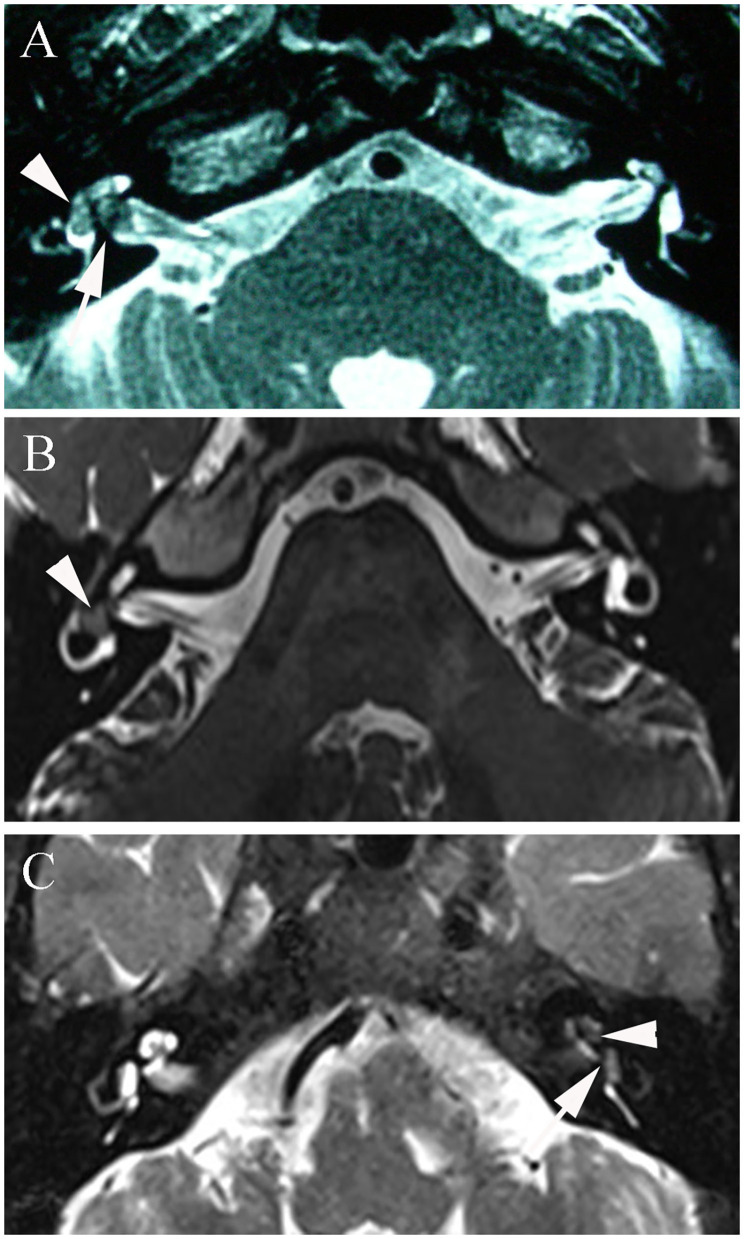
(A) T2-3D-space MRI of patient 1 with a transmacular tumor shows a hypointense mass in the vestibule (arrowhead) and a larger defect in the fundus of the internal auditory canal (arrow). (B) T2-3D-space MRI of patient 4 with an intravestibular tumor shows an isolated mass in the right vestibule (arrowhead). (C) Patient 7 with a vestibulocochlear tumor shows filling defects in both the vestibule (arrowhead) and cochlea (arrow) on T2-weighted MRI.
